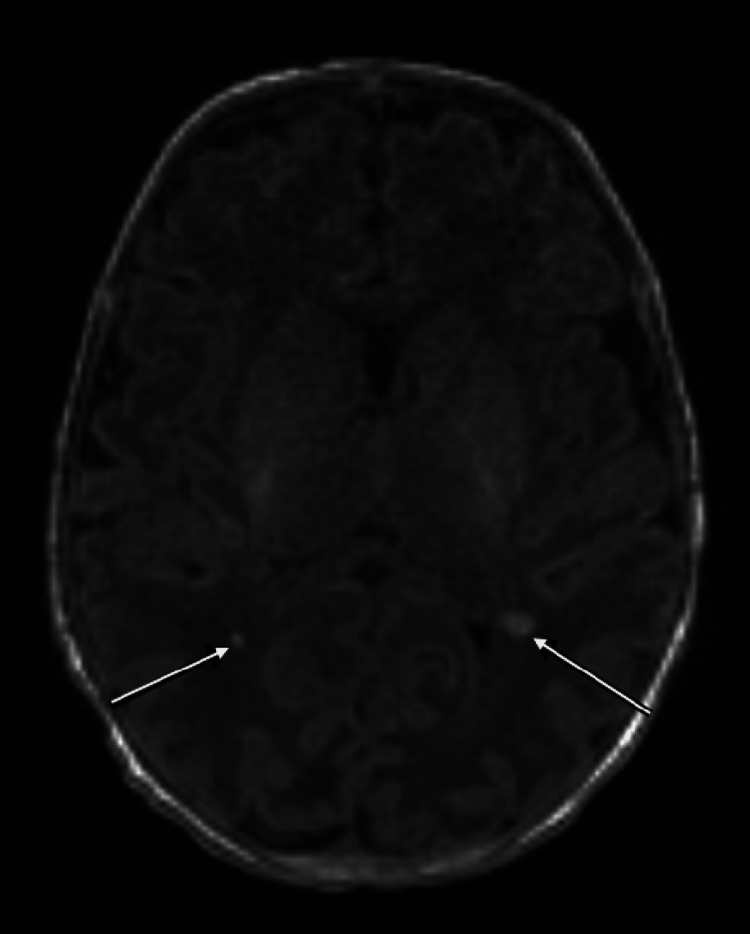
T1-weighted axial MRI image of the brain without the administration of intravenous contrast agent shows foci of hemorrhage in parietal white matter bilaterally (arrows).
